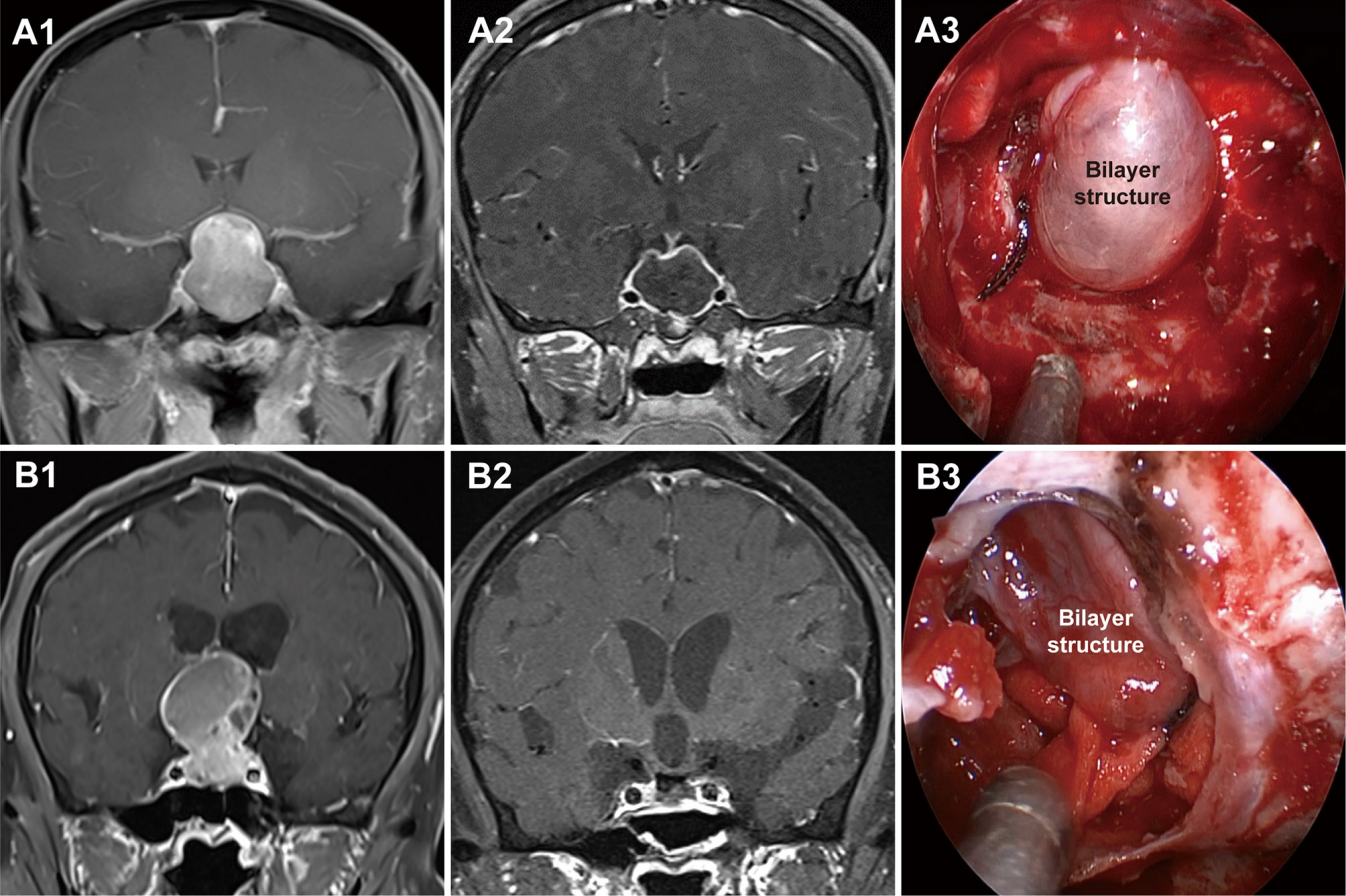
Two cases with grade 1a pituitary adenomas (PAs). (A1, B1) Preoperative, coronal post-gadolinium magnetic resonance image (MRI) showing grade 1a tumor with a regular smooth shape and clear border. (A2, B2) Postoperative MRI showing gross total resection. (A3, B3) Endoscopic views showing an intact bilayer structure formed by the DS and DS-attached residual pituitary gland.
